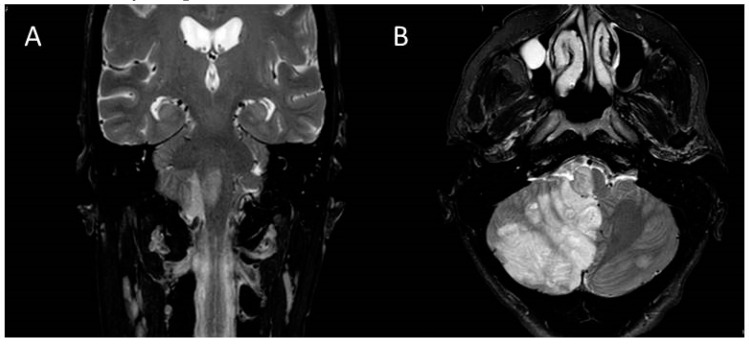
Coronal (A) and (B) transversal T2-weighted magnetic resonance imaging (MRI) which depicts ischemic stroke in the right cerebellar hemisphere, the upper medulla oblongata and the pontomedullary junction.
